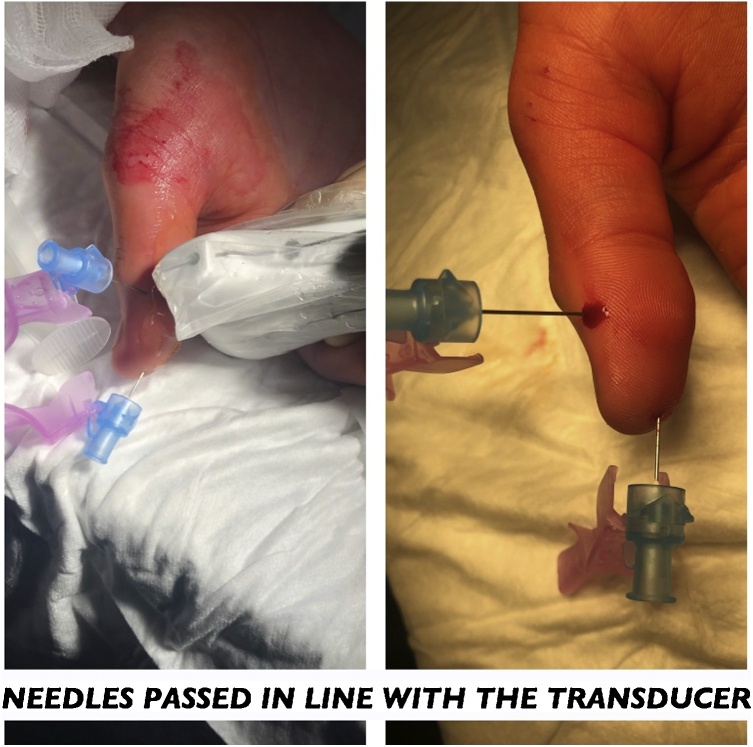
Insertion of orthogonal needles under ultrasound guidance, with needle tips just deep to the FB.
